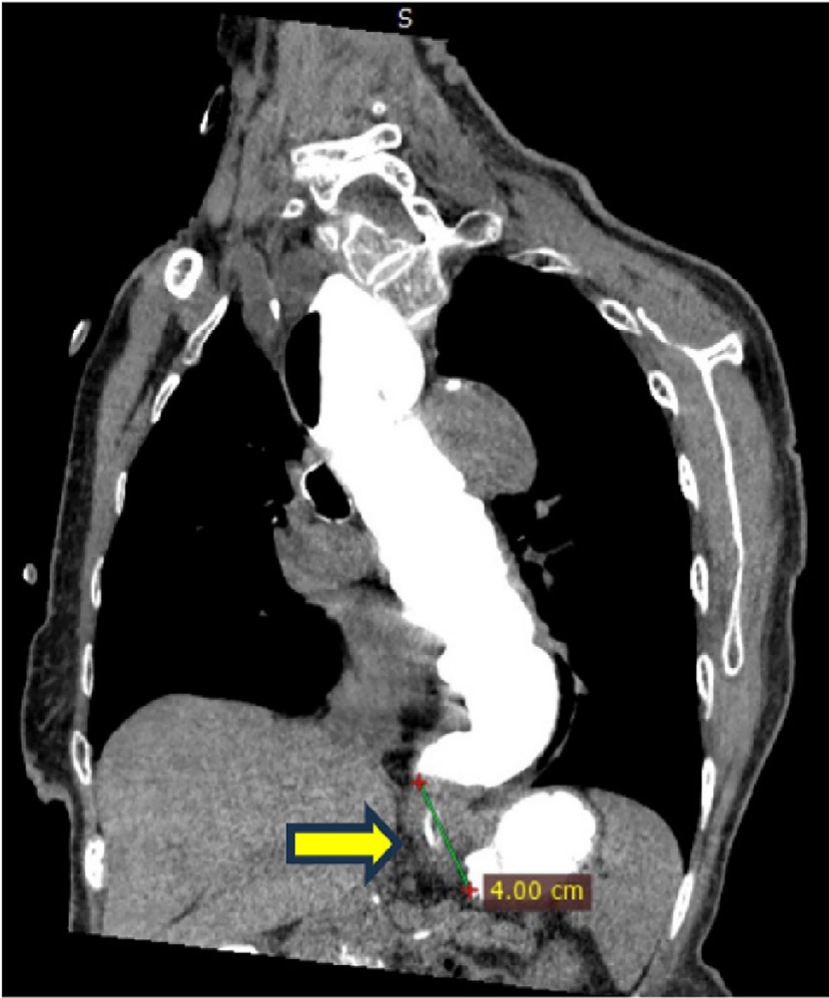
Distal esophageal stricture shown on contrast computed tomography (CT) scan.
